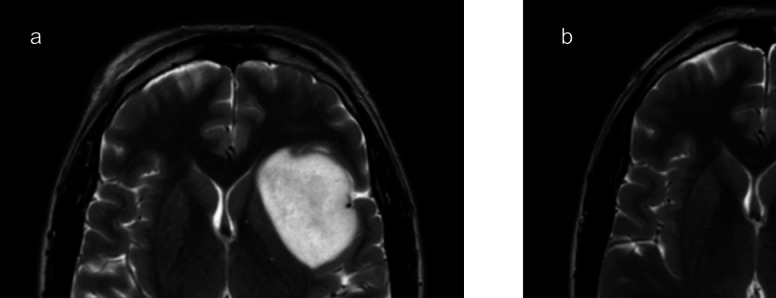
Case 2 – Preoperative MRI (axial view) demonstrated a left insular tumour (a). Post-operative MRI revealed a small volume of residual tumour in the posterior insula (b)
